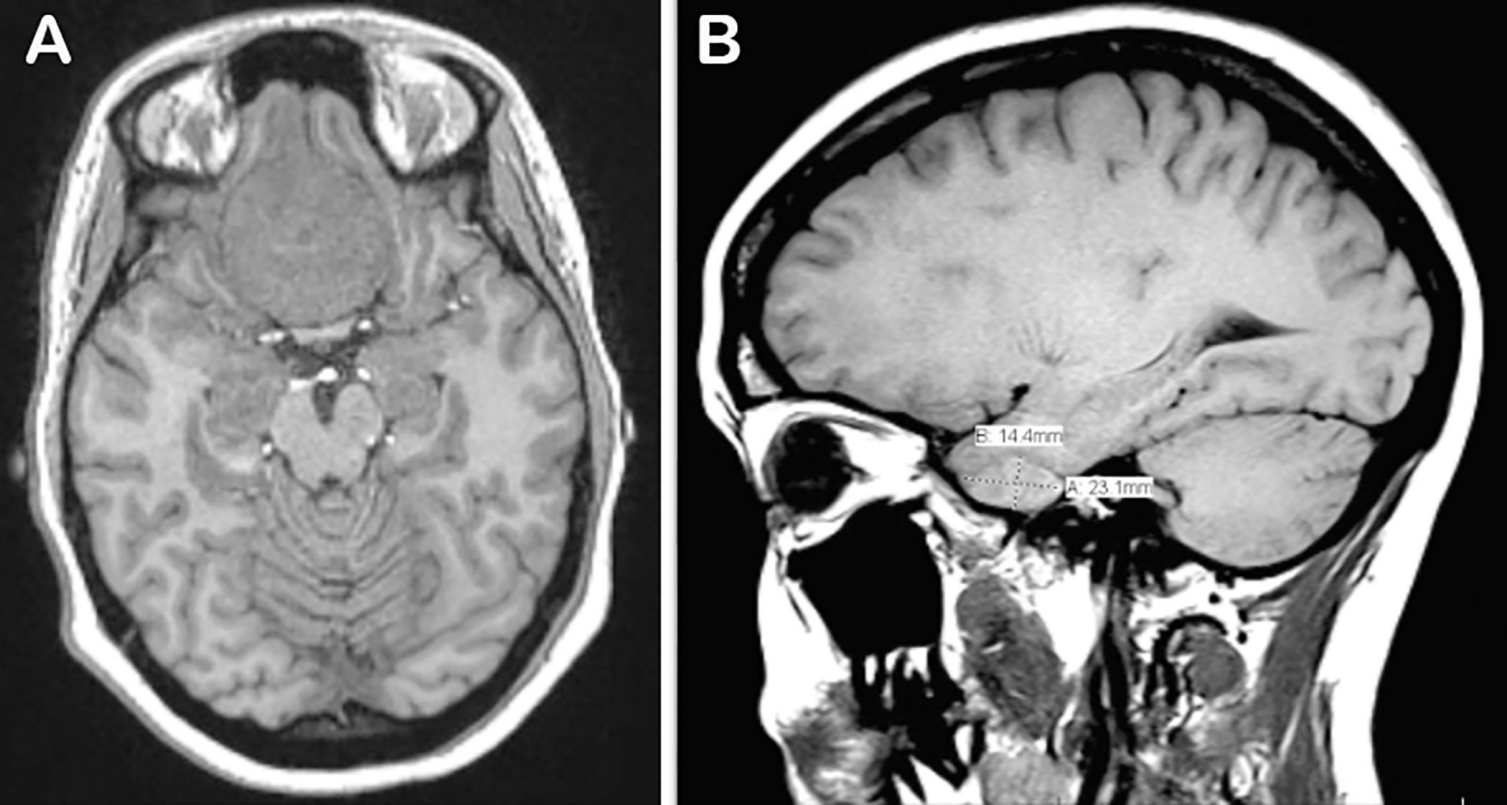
Pre-operative images. A) Axial T1 MRI revealed a large extra-axial meningioma within the anterior cranial fossa over the tuberculum sella with mass effect on optic chiasm and the prechiasmatic optic nerves. B) Sagittal T1 MRI revealed small extra-axial meningioma along right medial sphenoid wing.
